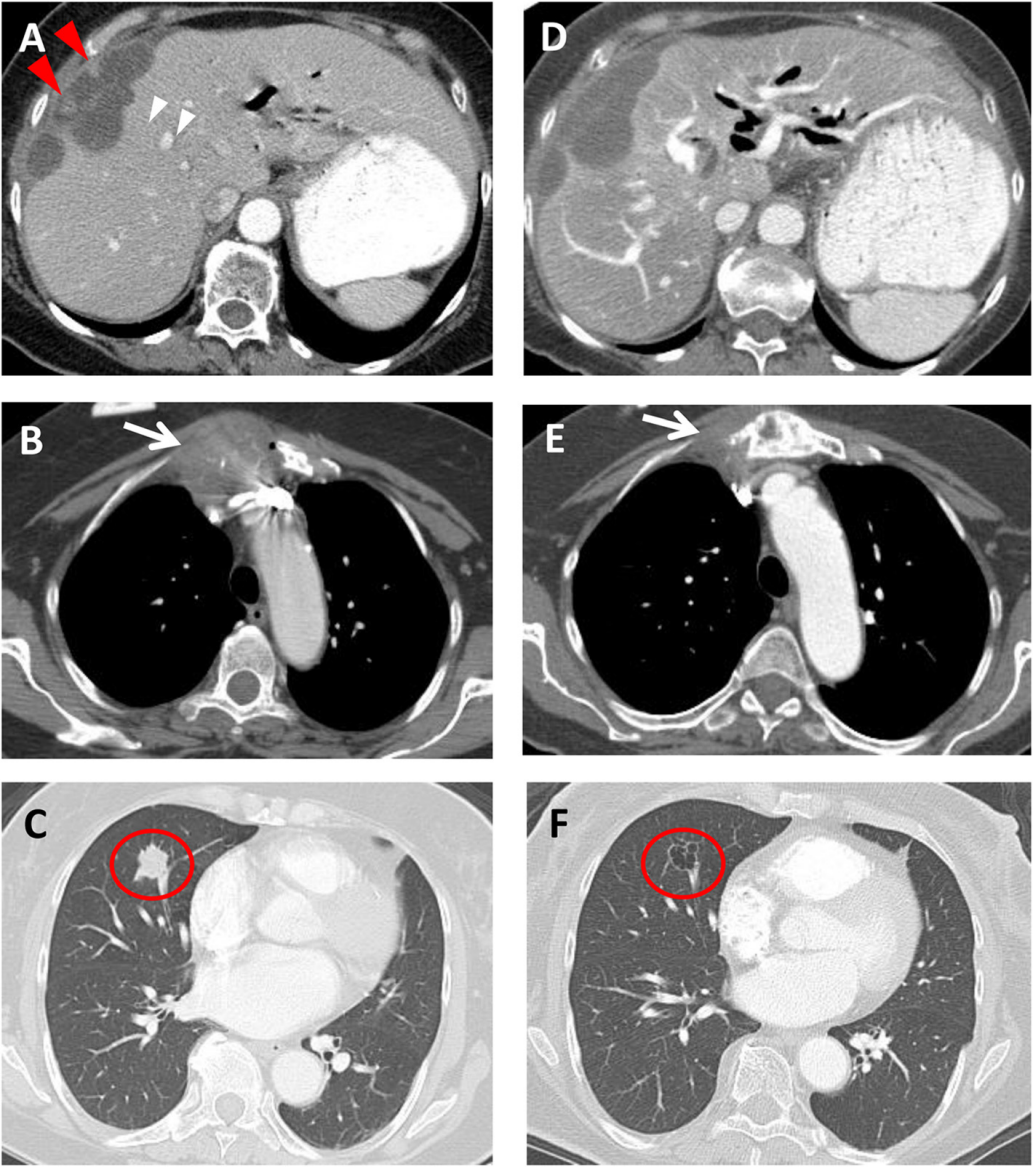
A 62-year-old female with recurrent metastatic gallbladder carcinoma with carcinomatosis. Axial contrast-enhanced CT images demonstrate a enhancing perihepatic nodules (*arrowheads*), b a 4.8-cm metastasis destroying the sternum (arrow), and c a 1.8-cm lung metastasis (*circle*). After 3 months of trastuzumab, d the perihepatic nodules are no longer visualized. e The sternum has become sclerotic with decreased size of the metastasis (*arrow*), and f the lung metastasis has undergone cavitation (*circle*).
